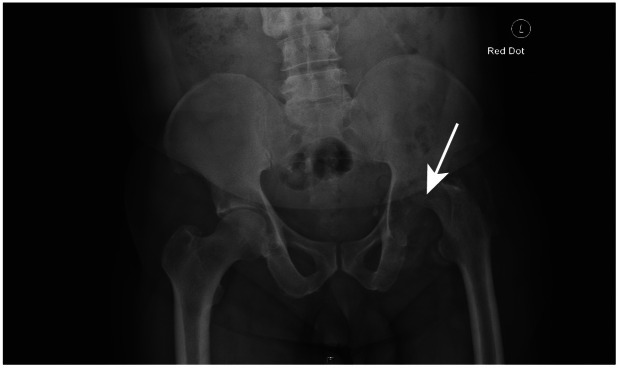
Plain film radiography of the pelvis.
